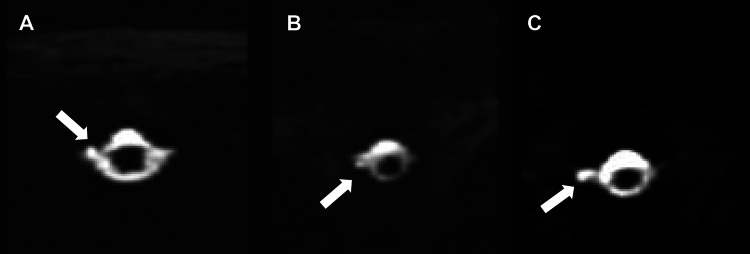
Axial reformatted MRI thoracic spine myelogram. Arrows show right-sided perineural cysts within the neuroforamina of (A) T4-T5, (B) T7-T8, and (C) T9-T10.
